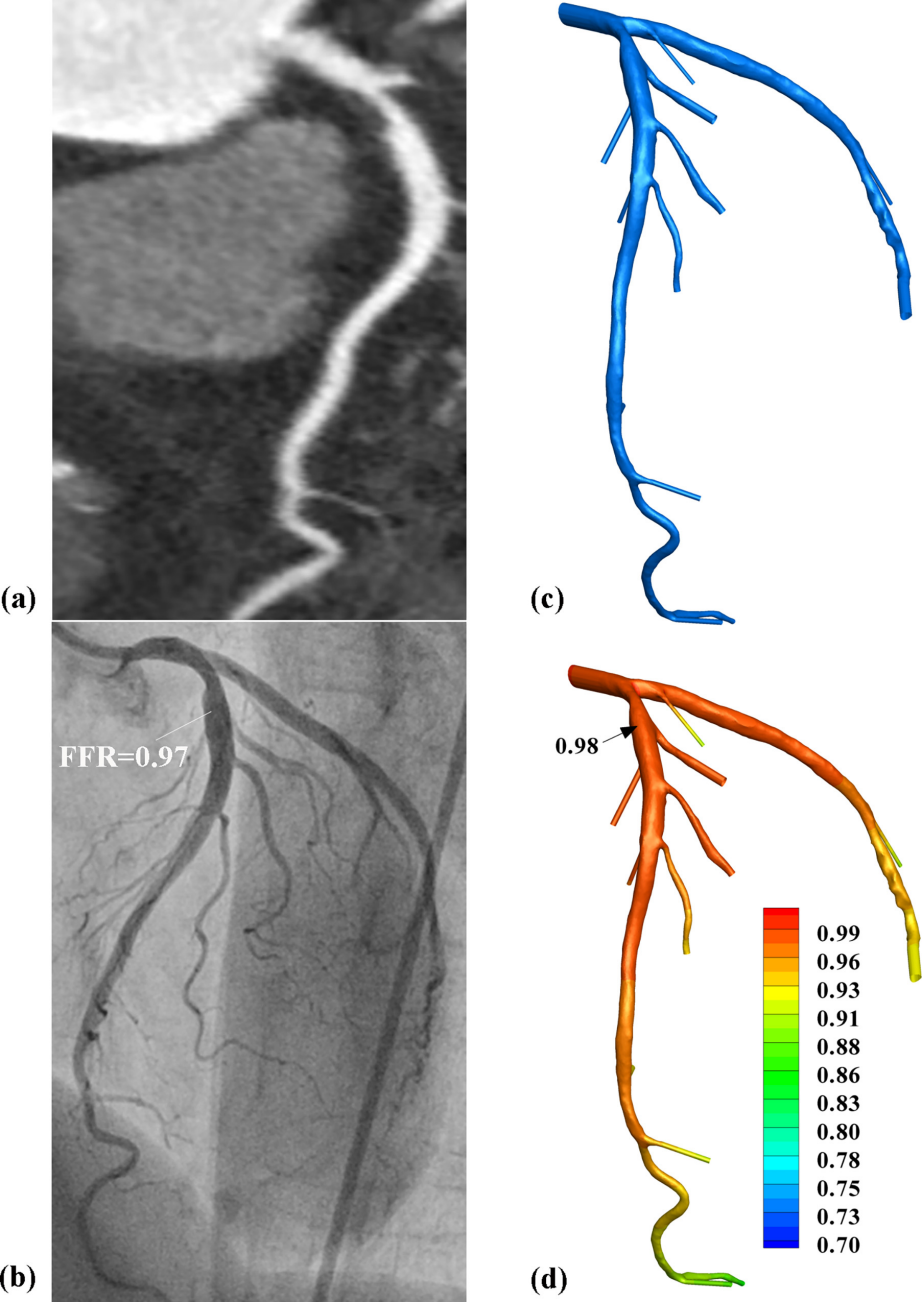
Representative patient with functional non-significant stenosis. Moderate luminal stenosis was observed from (a) CTA images and (b) invasive angiography measured FFR at 0.97 (FFR>0.8). Based on the (c) 3D model reconstructed from CTA images, CFD derived (d) pressure distributions on the 3D model and the calculated FFR_SS_ was 0.98.
